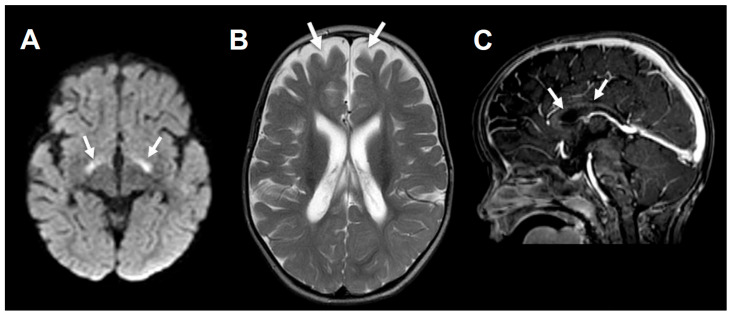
MRI brain scan of the female presented in Case C. At age one year the images display: (A)—diffusion-weighted imaging: bilateral restriction in the globus pallidus (arrows); (B)—T1-weighted imaging with gadolinium: thinned corpus callosum (arrows); (C)—T2-weighted imaging: moderate ventricular enlargement of the extraxial space (arrows) with mild ventricular dilatation.
